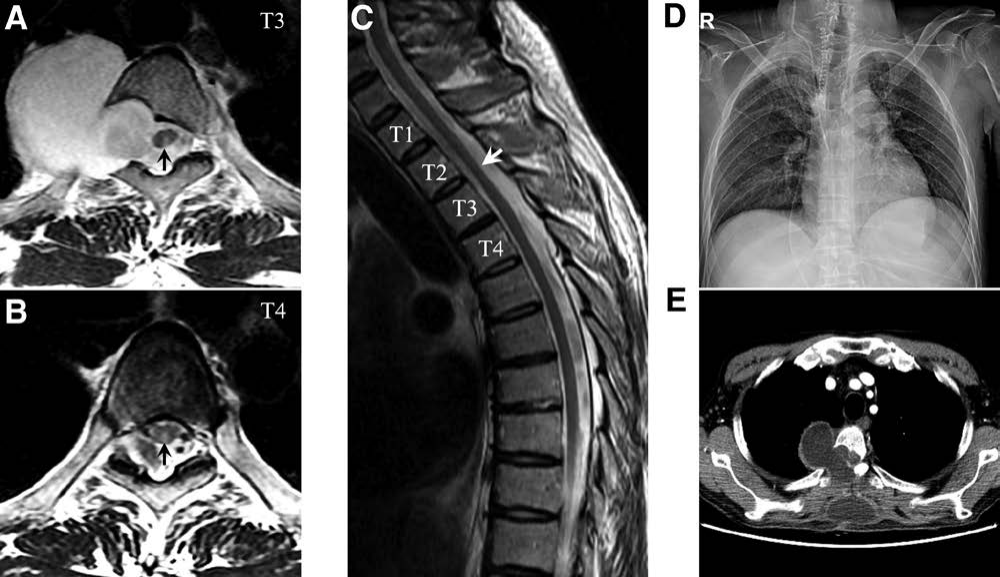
Follow-up radiologic finding 9 mo after the onset. Axial T2-weighted MRI shows focal hyperintensity (black arrows) involving the dorsal column of the spinal cord at the vertebral level of T3-4 (A and B). Midsagittal T2-weighted MRI shows spinal cord atrophy (white arrow) at the vertebral levels T2-T3 (C). Chest X-ray after decompression surgery shows no more oval opacity (D). Chest CT shows interconnected two peripherally-enhancing fluid collections in the paravertebral area and upper back (E). CT = computed tomography, MRI = magnetic resonance imaging.
